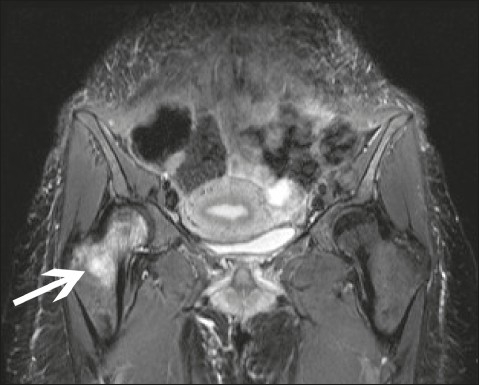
MRI 3 months later showing significant regression of the right hip edema.
